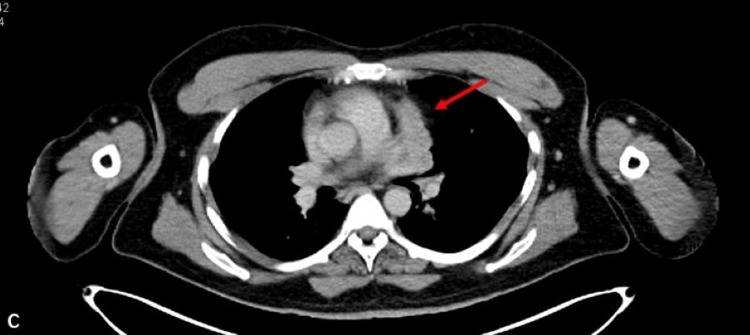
Axial contrast-enhanced computed tomography (CECT) of the chest at the level of the pulmonary artery
